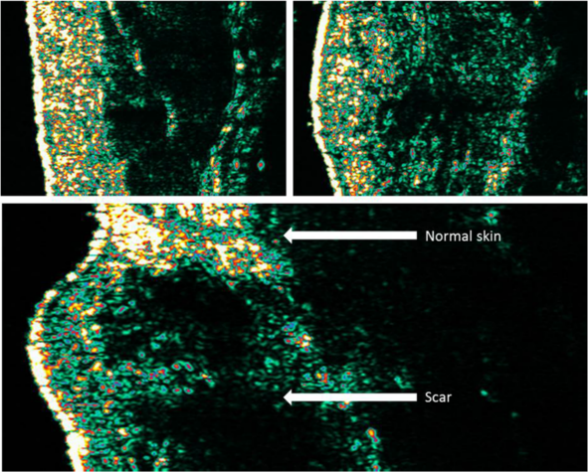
High-frequency ultrasound image of normal skin (*top left*, site: forearm). High-frequency ultrasound image of hypertrophic scar (*top right*, site: shoulder). High-frequency ultrasound image of normal skin (*top*) and adjacent scar tissue (*bottom*) (*bottom*, site: shoulder). Note that the scars appear more hypo-echoic as it is more homogenic and thus appears darker. Colours represent the intensity of the acoustic signal with bright colours (*yellow*) representing high-intensity and darker colours (e.g. *green*, *black*) representing low-intensity areas. (Source: Kwang Chear Lee, taken with Dermascan C)
